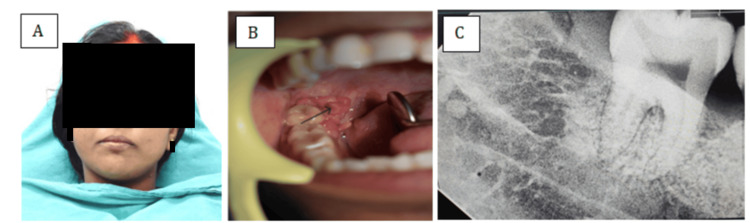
A) Extraoral image of the patient; B) Intraoral image of the patient (the arrow indicates the intraoral lesion); C) Preoperative intraoral periapical radiograph
